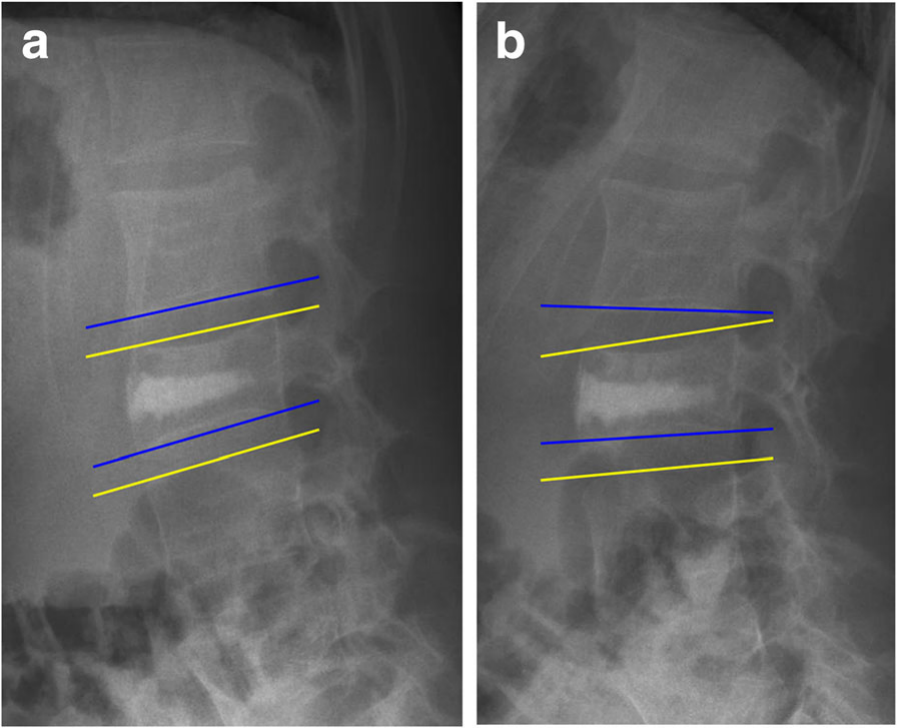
Flexion- (a) and extension (b) roentgenograms after removal of the instrumentation visualizing residual mobility
